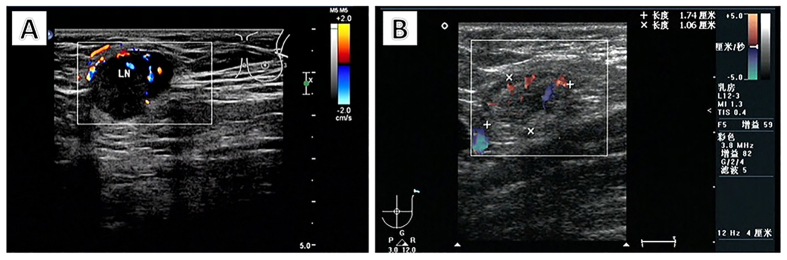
Ultrasound findings of positive and negative axillary lymph nodes. A positive lymph node (A) was detected in the left axilla with thickened cortex, and the hilum is disappeared. The diameters are 19 × 16 mm. A negative lymph node (B) was detected in the left axilla with normal cortex and hilum. The diameters of the lymph node are 21 × 6 mm.
